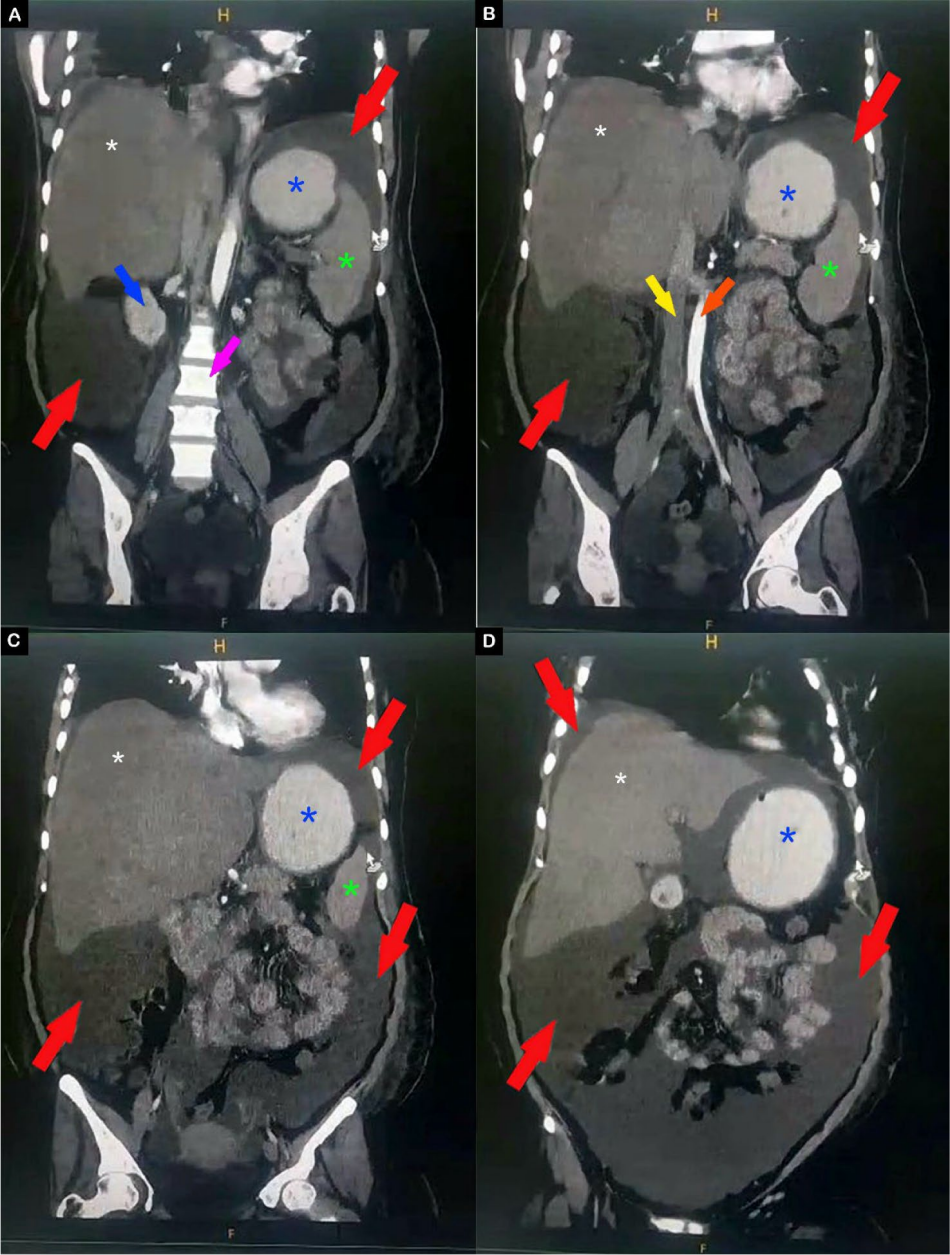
Coronal computed tomography sections of the abdomen showing massive ascites (red arrows). A Posterior coronal section showing the right kidney (blue arrow), lumbar vertebrae (pink arrow), liver (white asterisk), stomach (blue asterisk), and spleen (green asterisk). B Mid-abdomen coronal section displaying the inferior vena cava (yellow arrow) and the abdominal aorta (orange arrow). C Mid-abdomen coronal section demonstrating massive ascites surrounding the bowel loops (red arrows). D Anterior coronal section revealing a subphrenic fluid collection above the liver
